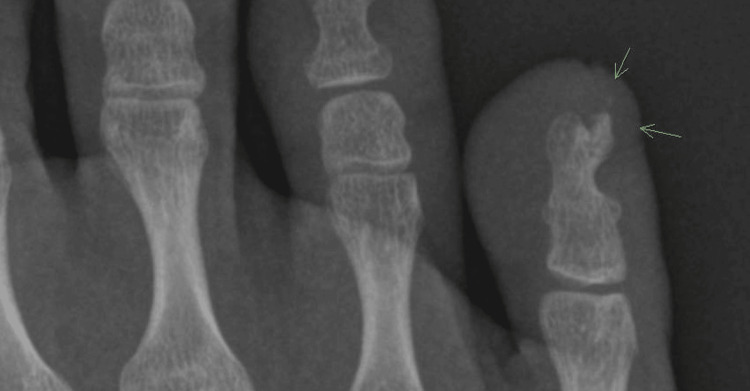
X-ray showing a radio-opaque mass consistent with a subungual exostosis. Arrow denotes the subungual exostosis arising from the distal phalanx
